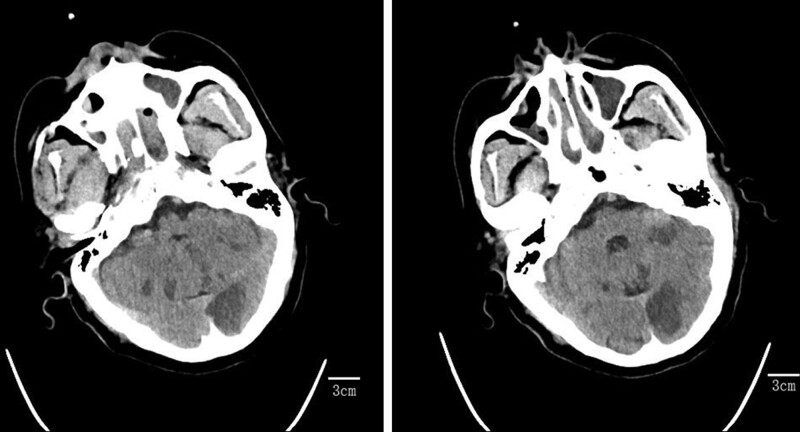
Head CT reexamined 24 hours after intravenous thrombolysis showed posterior circulation cerebral infarction. CT = computed tomography.
